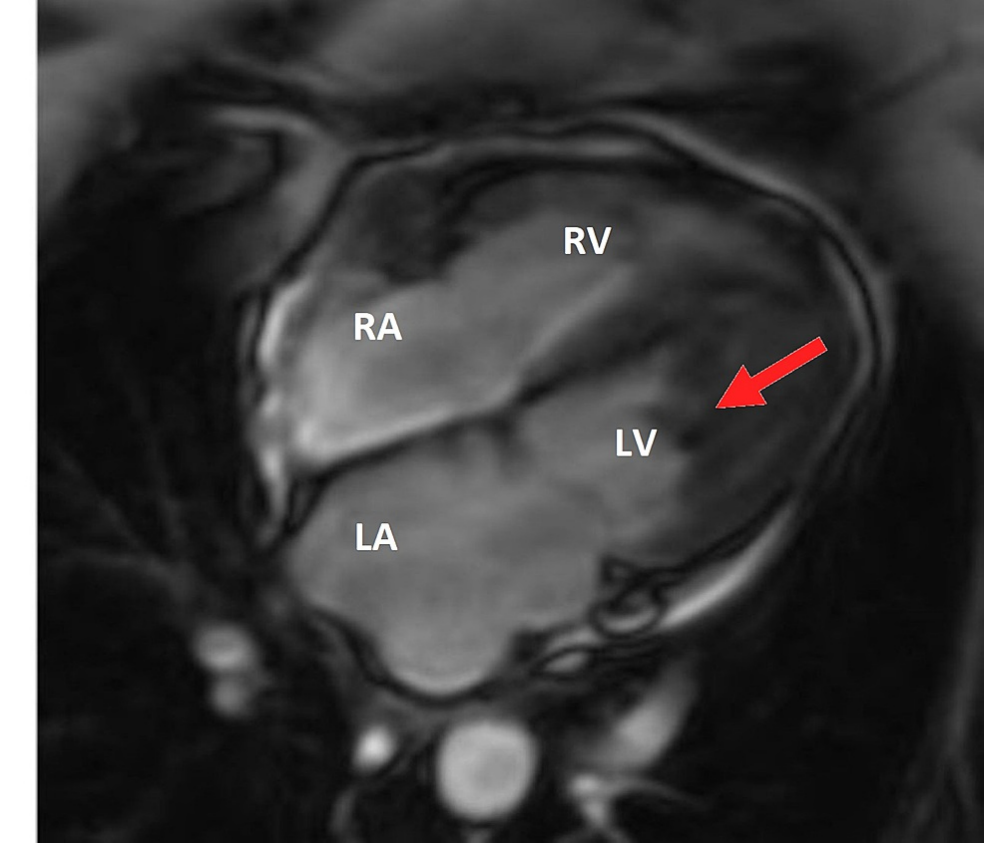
A cardiac MRI revealing a gigantic LV mass. LV, left ventricle; LA, left atrium; RV, right ventricle; RA, right atrium
